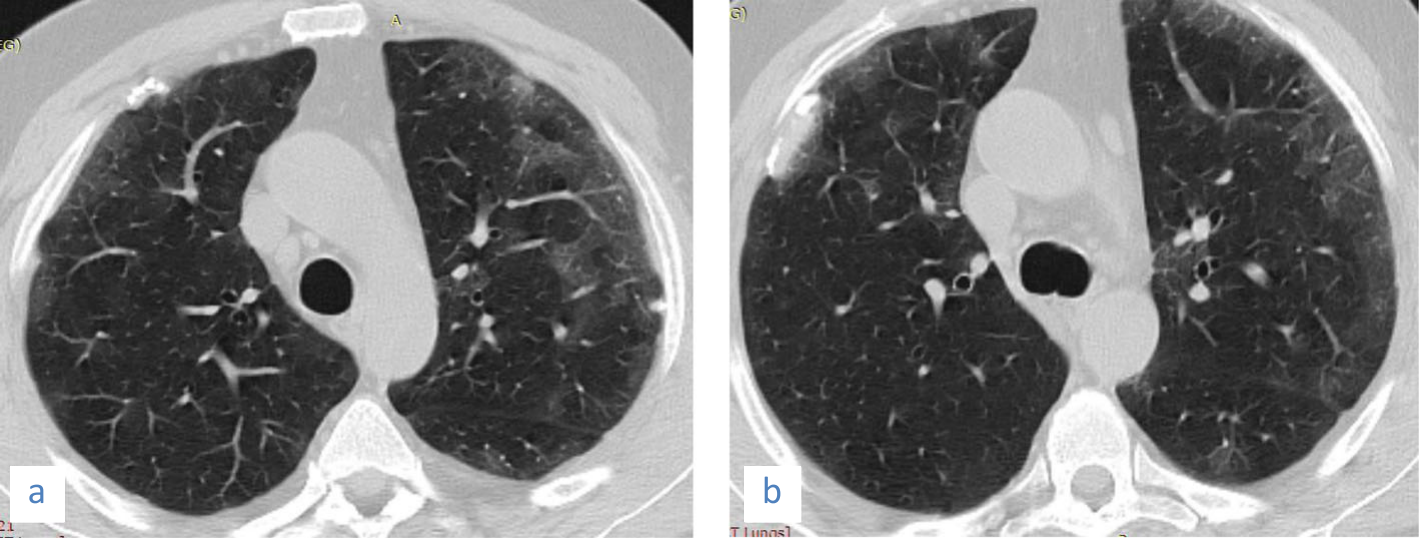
Male patient 56 years old with COVID-19. CT axial images (a) and (b) show bilateral predominantly peripheral rounded ground glass opacities with vascular thickening. Note the bilateral pleural plaques with calcification due to asbestos exposure
